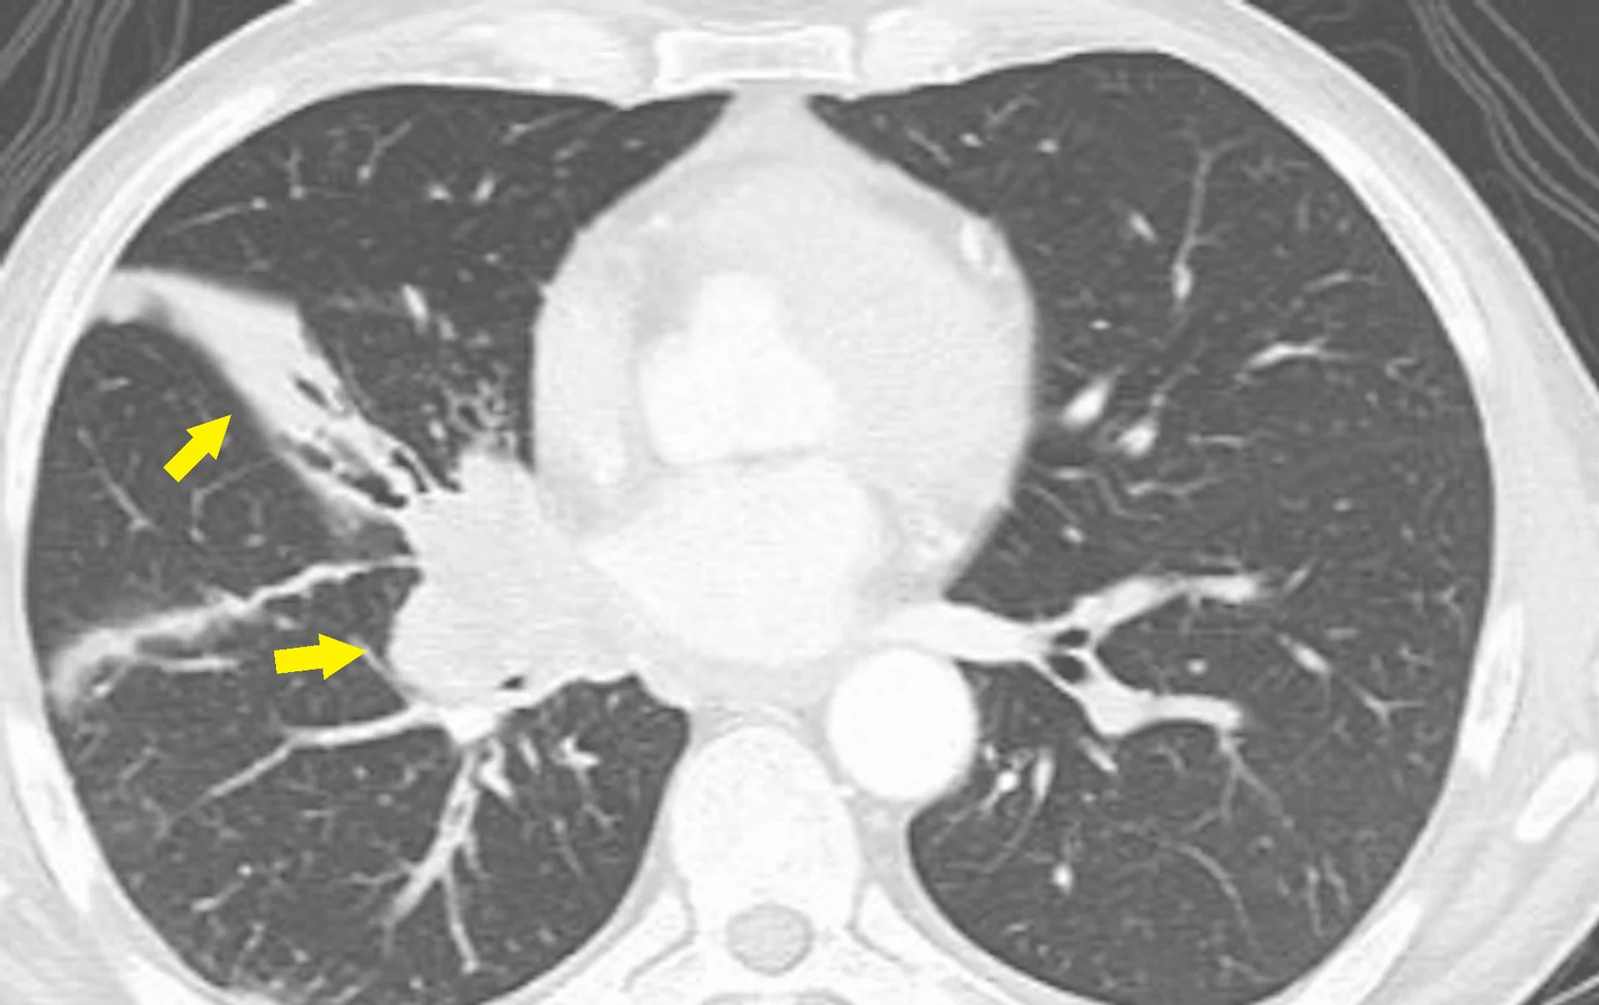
CT scan with IV contrast of the chest showing a right perihilar mass with associated bronchial obstruction and atelectasis The top yellow arrow indicates a hyperdense area of atelectasis in association with the obstructive bronchial mass indicated by the bottom arrow.
